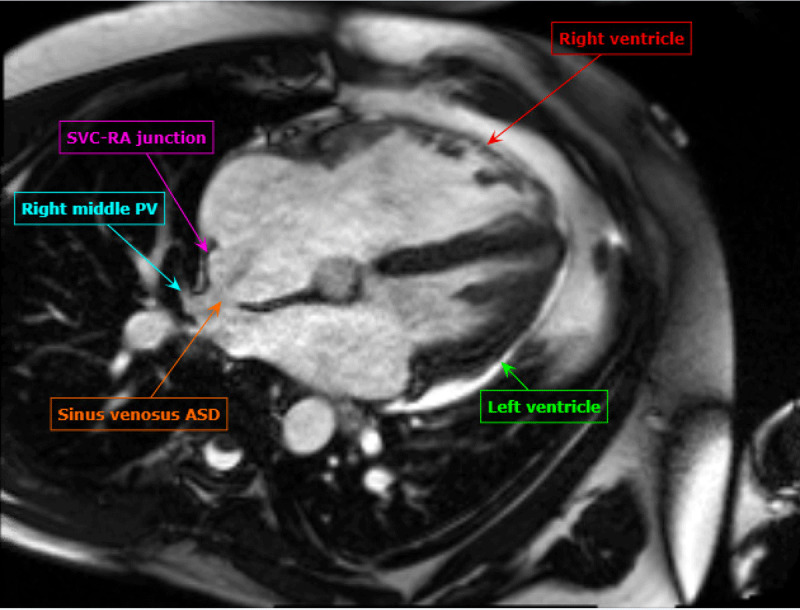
Four-chamber stack of cardiac magnetic resonance imaging showing sinus venosus atrial septal defect as labeled.
